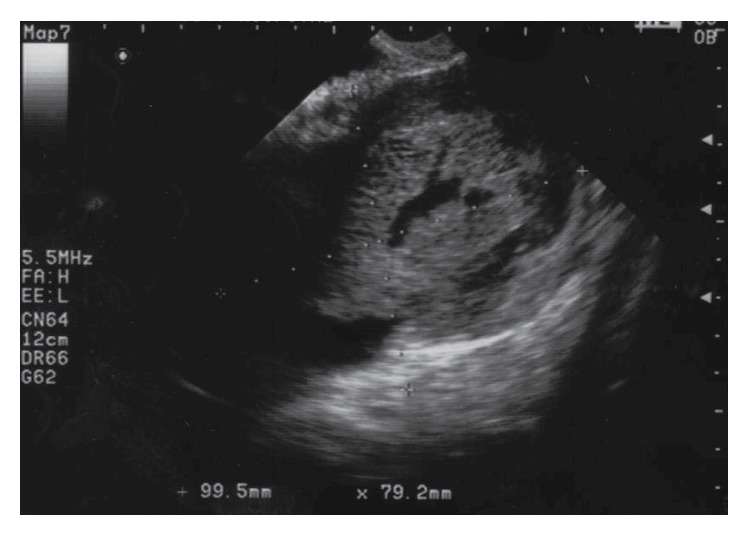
Transabdominal ultrasonographic image of the tumor. The mass was 10 cm in diameter, with a high echoic area in the center surrounded by a low echoic area.
